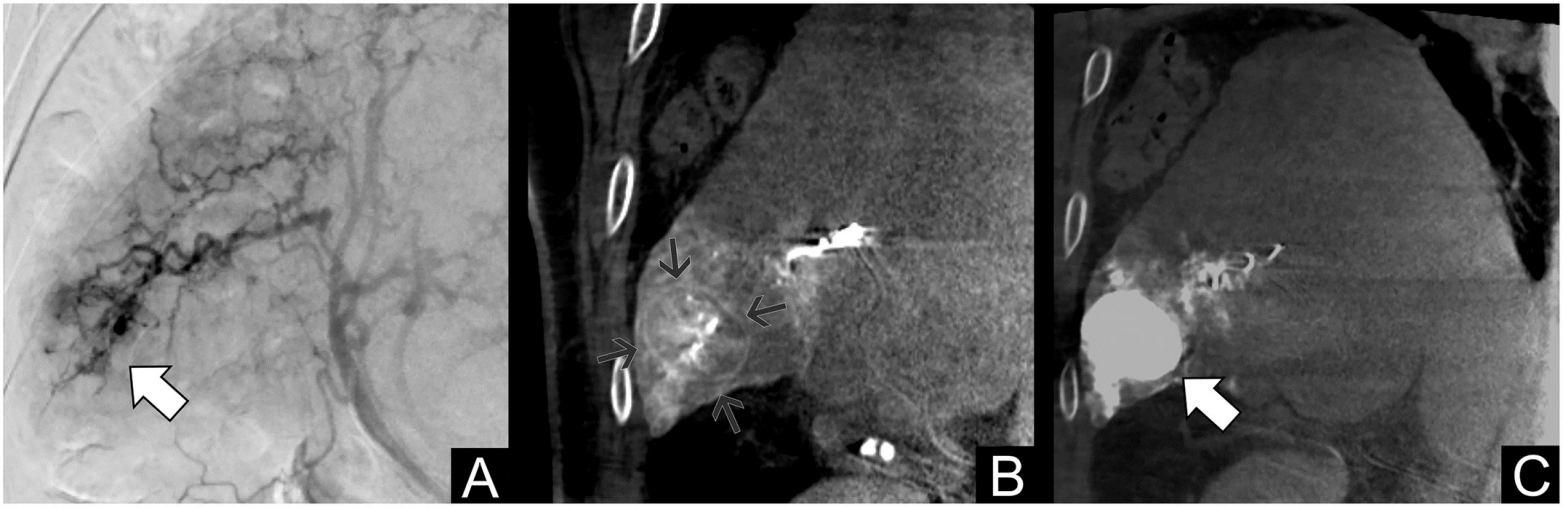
Patient #130, treated with TAE for multifocal HCC at 68 years (BCLC B, HCV+). A: Digital subtraction angiography of the right hepatic artery showing an HCC nodule in the sixth segment. B: Cone-Beam CT (coronal reconstruction) during contrast injection, highlighting the nodule capsule. C: Non-contrast final control after TAE showing marked contrast retention inside the lesion and complete arterial blood flow stoppage. The patient died during observation, with a survival of 21.1 months.
